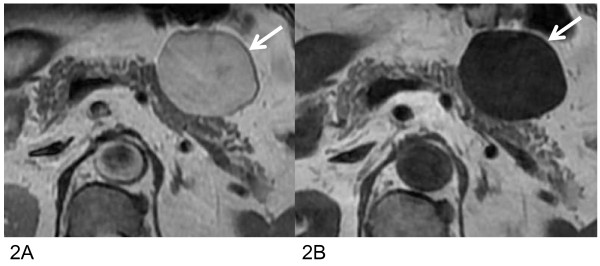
Magnetic resonance imaging results. (a) Axial T_2_-weighted MR image shows that the mass has almost homogeneous high signal intensity, (b) Axial T_1_-weighted MR images show that the mass has homogeneous low signal intensity.
